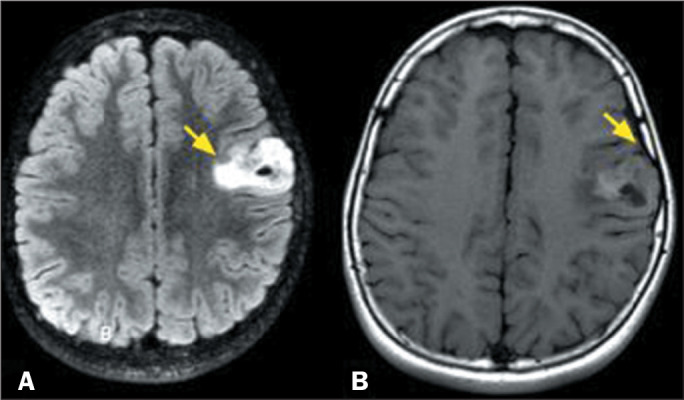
MRI scans of a 16-year-old male patient. A FLAIR sequence (A) showing
